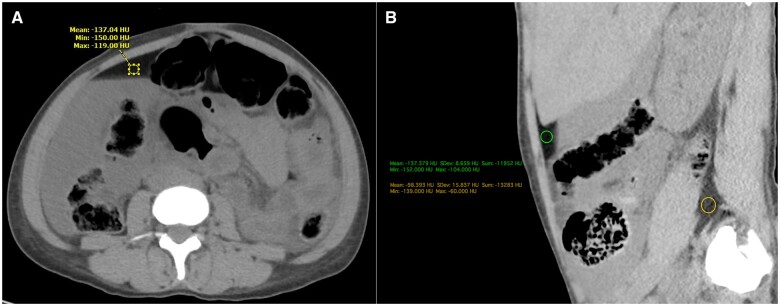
(A) Axial CT section at the level of the mid abdomen shows dependent high attenuation fluid, filling the right paracolic gutter, and non-dependent low attenuation fat towards the anterior abdominal wall, forming a fat-fluid level. A region of interest (ROI) in the non-dependent fluid, shows attenuation value of −137 Hounsfield Units (HU), suggestive of fat. (B)This non-dependent fluid has a lower attenuation value than the mesenteric or retroperitoneal fat (−98 HU). This fluid was consistent with the lipid laden peritoneal fluid that was found on laparotomy.
